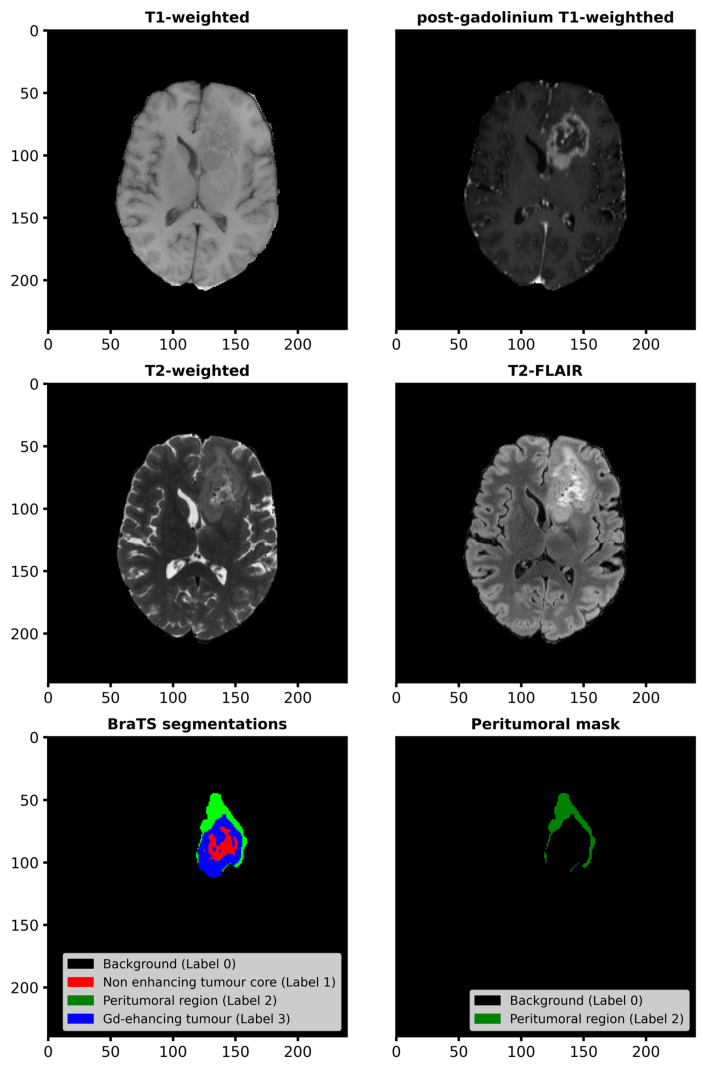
The images display slices from the T1-weighted and post-gadolinium T1-weighted sequences (top row), T2-weighted and T2 Fluid Attenuated Inversion Recovery sequences (middle row), along with the tumor segmentation (third row, left) and the extracted peritumoral region mask (i.e., edema - third row, right), used for radiomics analysis. Data are from subject BraTS-GLI-00000-000 in the BraTS 2023 challenge training set.
